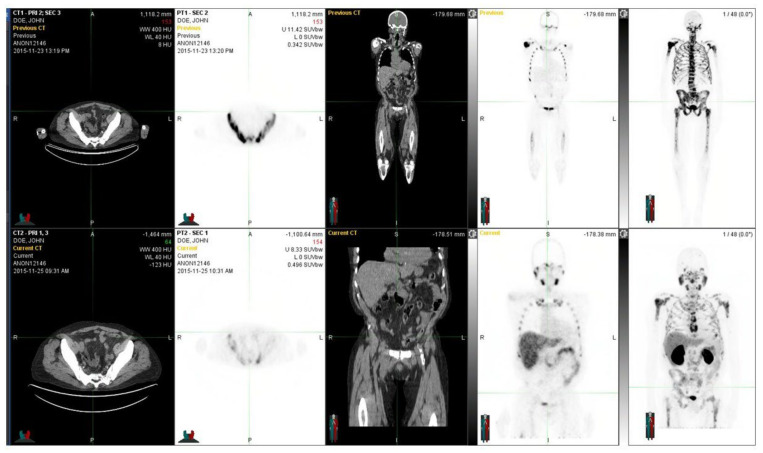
The figure demonstrates a 76-year-old patient with a T2N0M0 (GS 8) prostate cancer diagnosed more than 15 years earlier. He was originally treated with radiotherapy and androgen deprivation. After multiple relapses, an operation, additional radiotherapy, chemotherapy, and new drugs were introduced. At the time of the investigation, the widespread skeletal disease was observed both with NaF PET (upper row) and ^68^Ga-PSMA PET (lower row). There are no essential differences in their distributions. At the time of these images, the S-PSA was 293 (patient 14).
